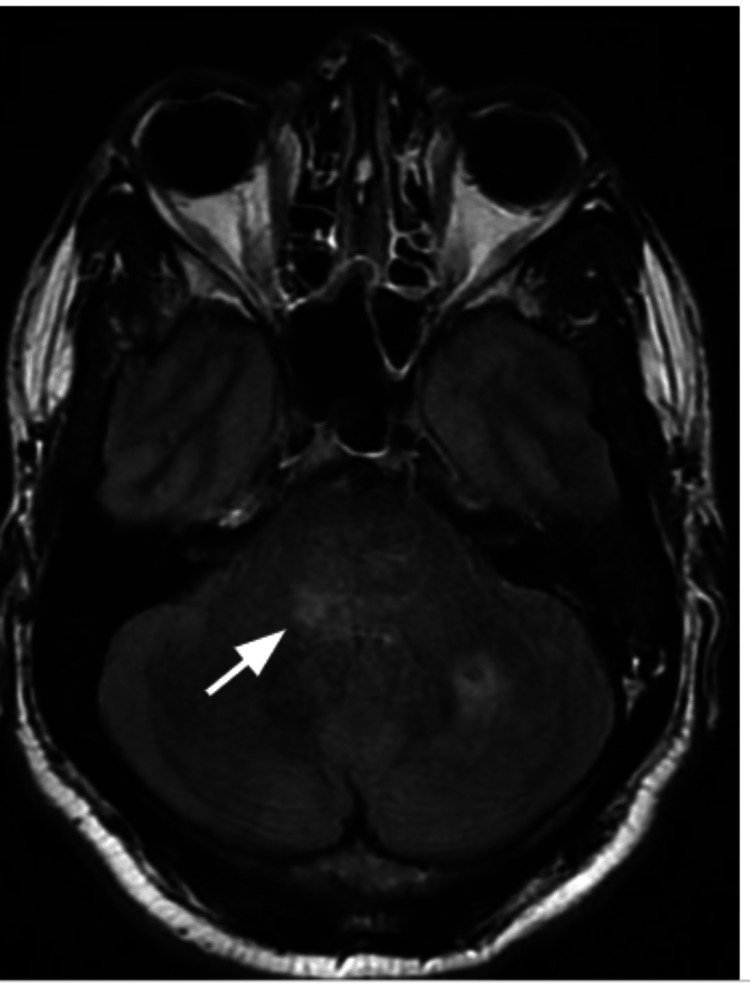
MRI brain FLAIR T2 weighted series showing edema in the pons
